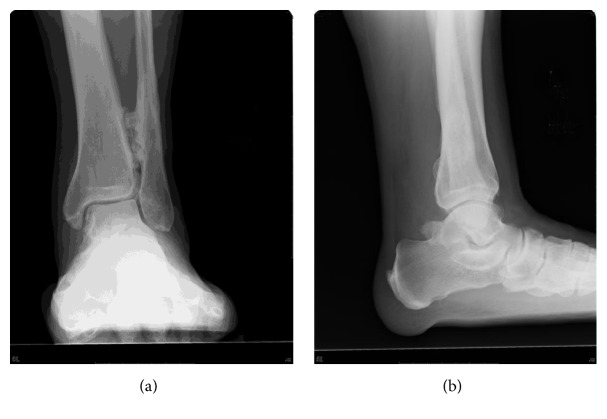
Preoperative radiographic findings. Anteroposterior (a) and lateral (b) views while standing upright.
